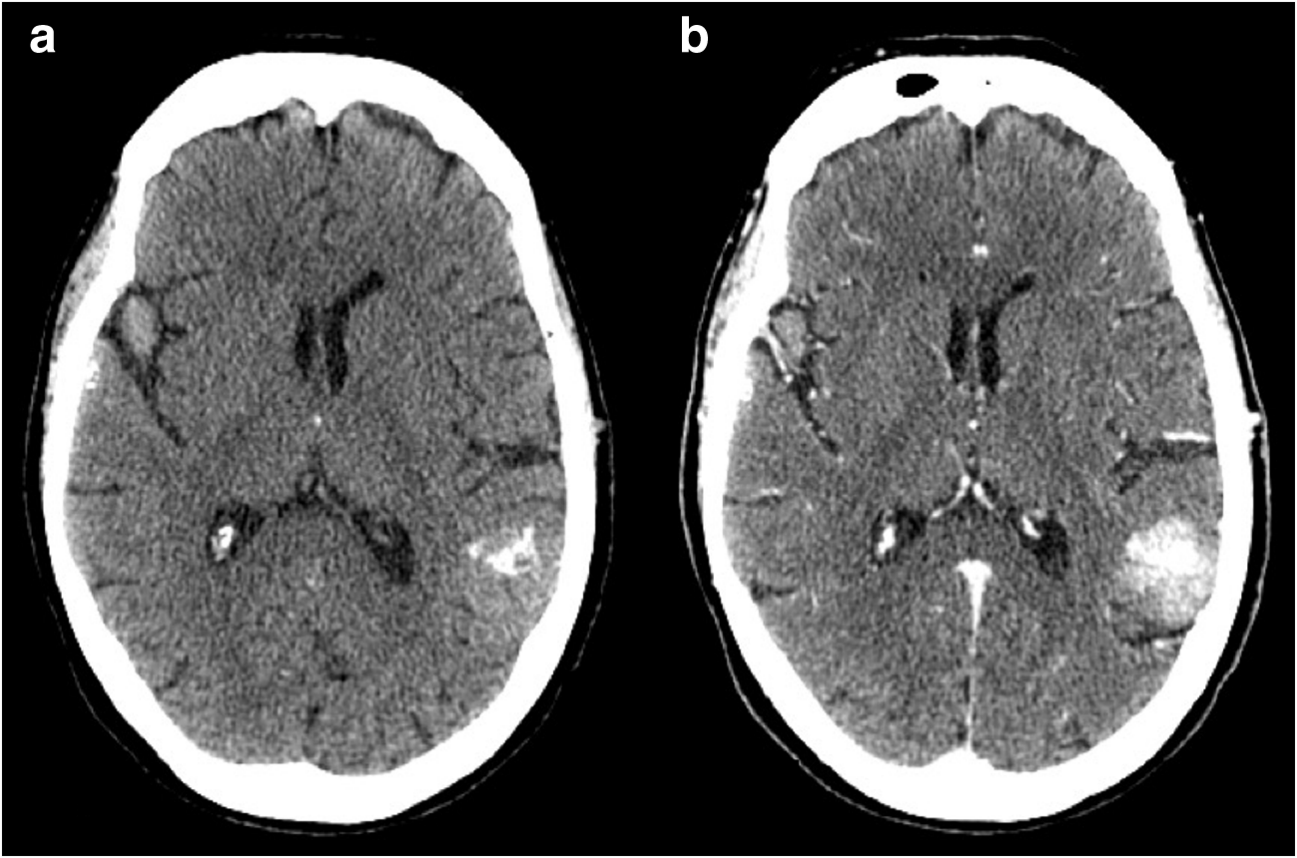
Ab initio CBM. Computed tomography, axial plane. a Pre-contrast CT scan with evidence of coarse brain calcification within a metastatic left temporoparietal intra-axial lesion, in a patient with a primary adenocarcinoma of the lung. b Post-contrast CT scan showing intense enhancement of the lesion. The patient, with multiple CBM (one of which can be partially seen in the right temporal lobe), did not undergo RT at the moment of diagnosis
